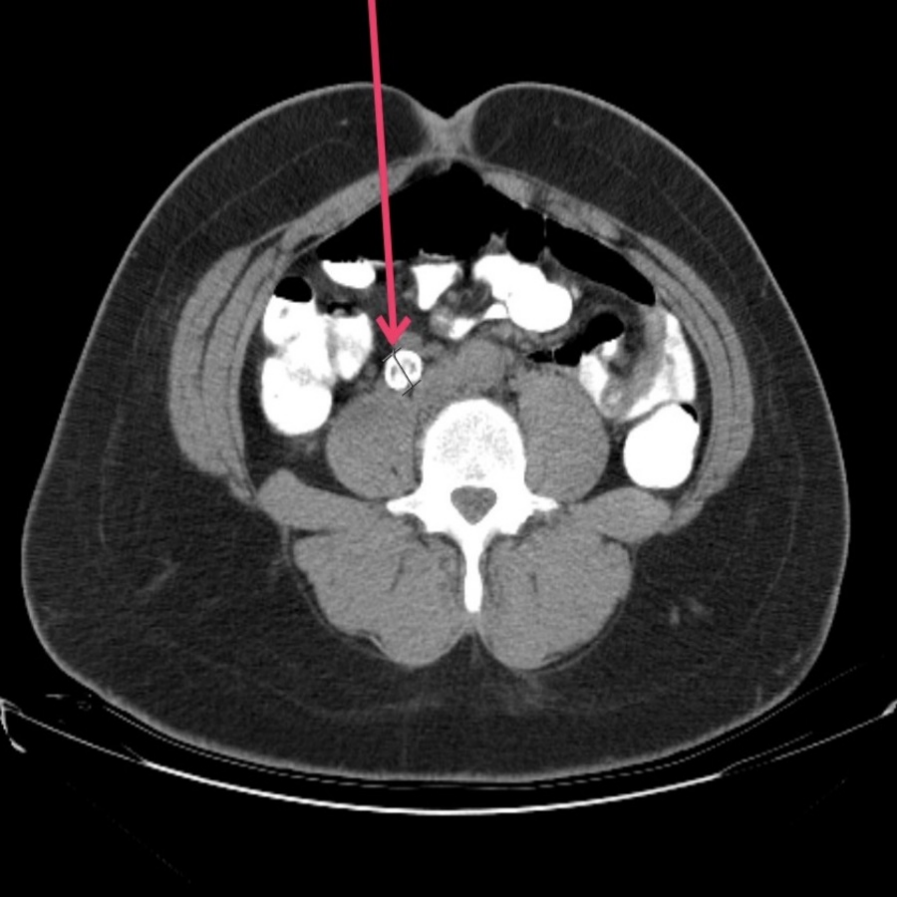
Computed tomography of the abdomen and pelvis Right retroperitoneal high density mass (red arrow) on the anterior surface of psoas muscle.
